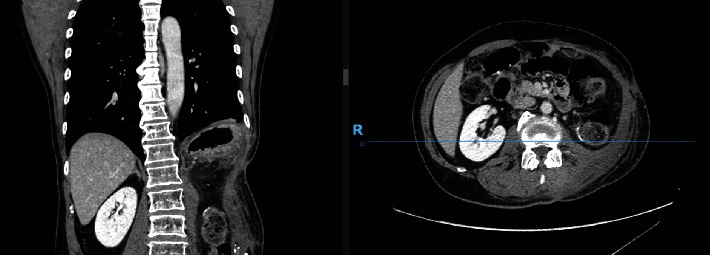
Postoperative CT scan of patient at 6-month mark. Note: CT scan of the lateral abdominal wall reconstruction at 6 months postoperatively.
